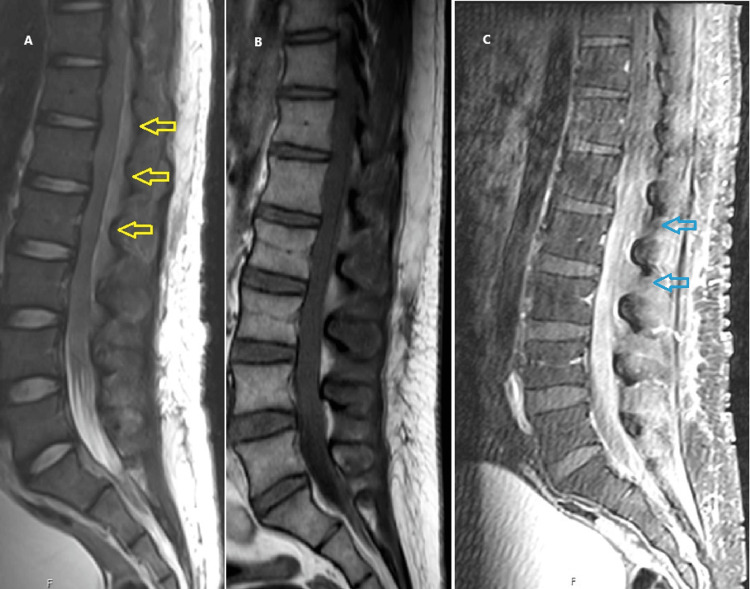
Sagittal sections from a contrast enhanced MRI of the lumbar spine showed a circumferential expansion of the epidural space from T11 to L4 (T1 hypointense and intermediate T2 signal with slight enhancement). This was noted to be exerting mass effect upon the thecal sac. There was also subtle enhancement of the nerve roots. A. Sagittal T2-weighted sequence (yellow arrows indicate epidural space expansion). B. Sagittal T1-weighted sequence. C. Sagittal post-contrast T1-weighted sequence (blue arrows indicate nerve root enhancement).
